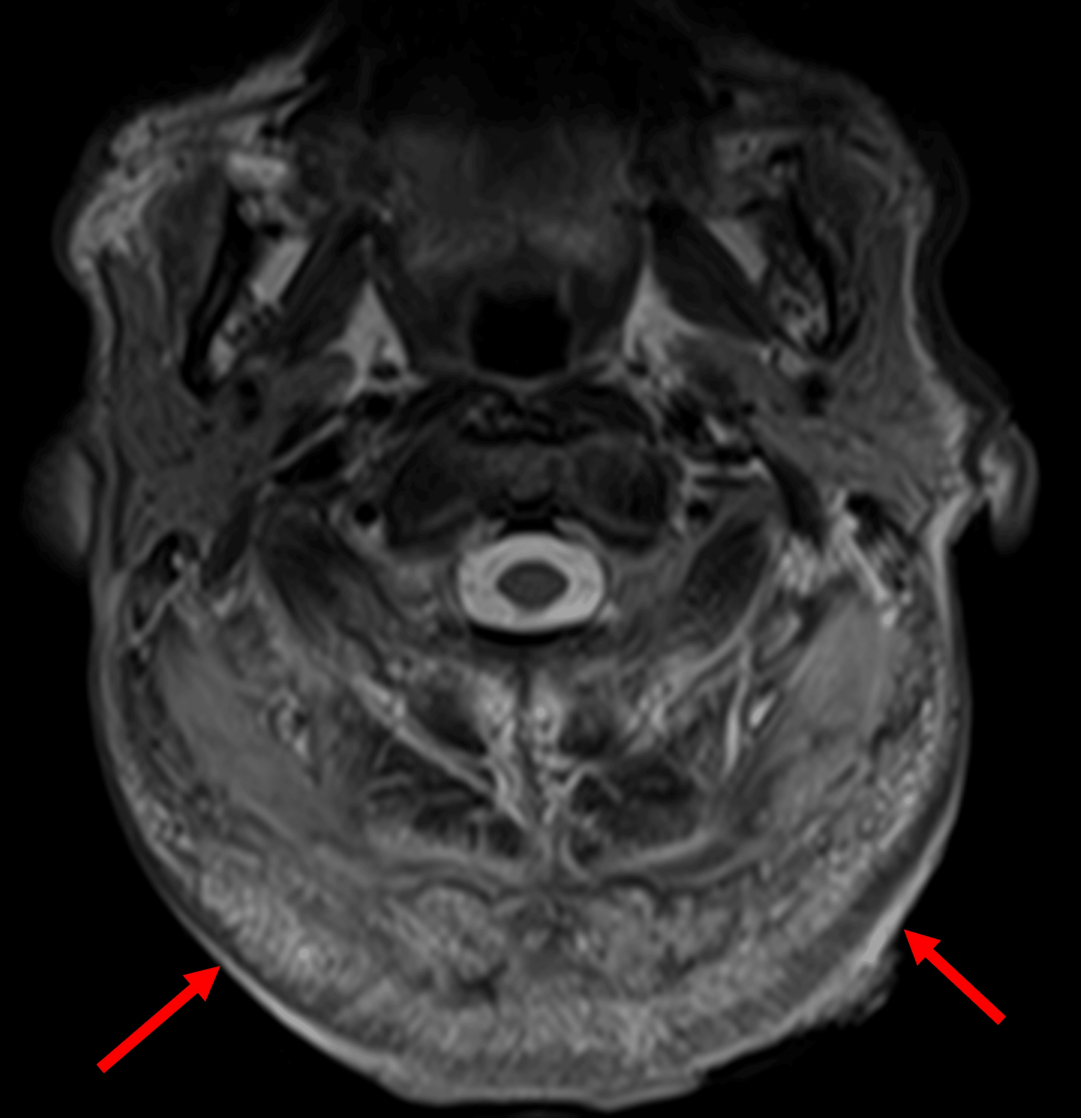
Preoperative MRI T2-weighted magnetic resonance imaging (MRI) revealed a high-signal area extending to the muscle layer Red arrow: tumor
